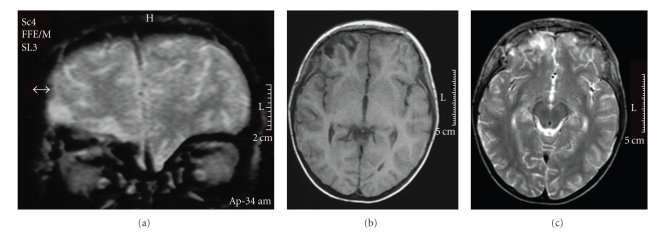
Magnetic Resonance Imaging (MRI) performed 2 weeks after trauma: FFE-coronal (a), T1 weighed (b), and T2-axial (c) sections showed bilateral (right > left) basi-frontal lesions. Right insular lesion is not shown.
